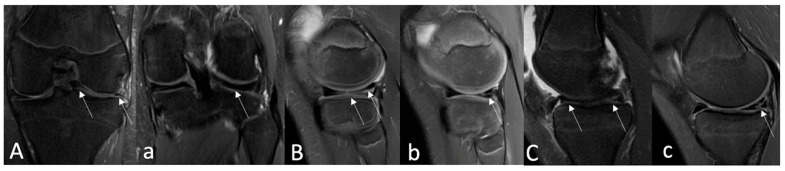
Examples of postoperative MRI assessment based on Stoller and Crues’ three-stage classification. (A)—Preoperative coronal PD-FSE, FS MR image shows (arrows) a bucket handle-type lateral meniscus tear in a 16-year-old boy. Twelve-month postoperative coronal (a) MR image shows a repaired lateral meniscus and no intrameniscal signal alterations (arrow)—classified as MRI Grade I using Crues and Stroller classification. (B)—A preoperative sagittal PD-FSE, FS MR imaging of an 11-year-old boy reveals (arrows) a complex (radial + longitudinal)-type lateral meniscus tear. Sagittal PD-FSE, FS (b) MR image obtained 12 months after surgery, where sagittal PD-FSE, FS (b) indicates (arrow) a partially healed lateral meniscus with persisting intrameniscal signal changes that do not extend into the joint space—classified as MRI Grade II using Crues and Stroller grading. (C)—A 14-year-old girl’s preoperative sagittal PD-FSE, FS MR imaging demonstrates (arrows) a medial meniscus tear of the bucket-handle type. Fourteen-month postoperative sagittal (c) PD-FSE FS MR images establish (arrow) an unhealed medial meniscus with signal changes extending within the joint space—classified as MRI Grade III using Crues and Stroller grading. MR—magnetic resonance; FSE—fast spin echo; FS—fat saturation.
